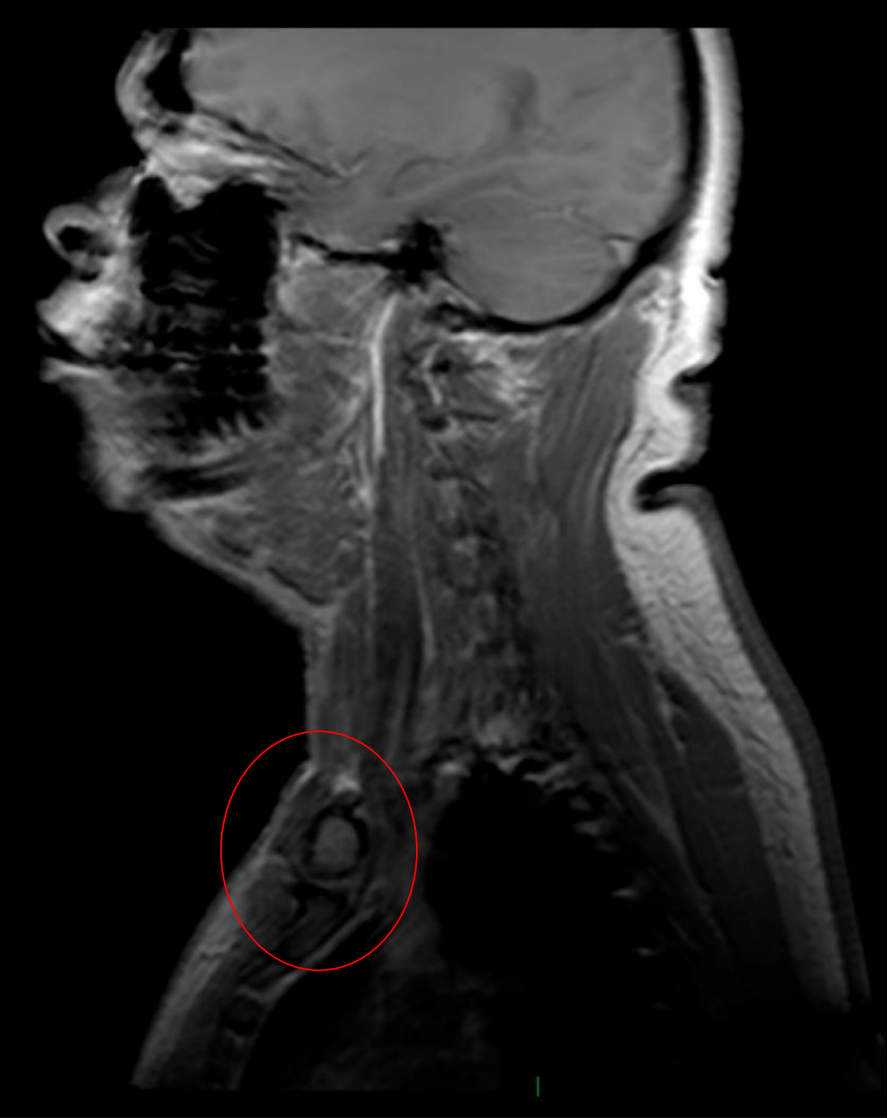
Magnetic resonance imaging showing partial tumor response after three months of imatinib treatment.
